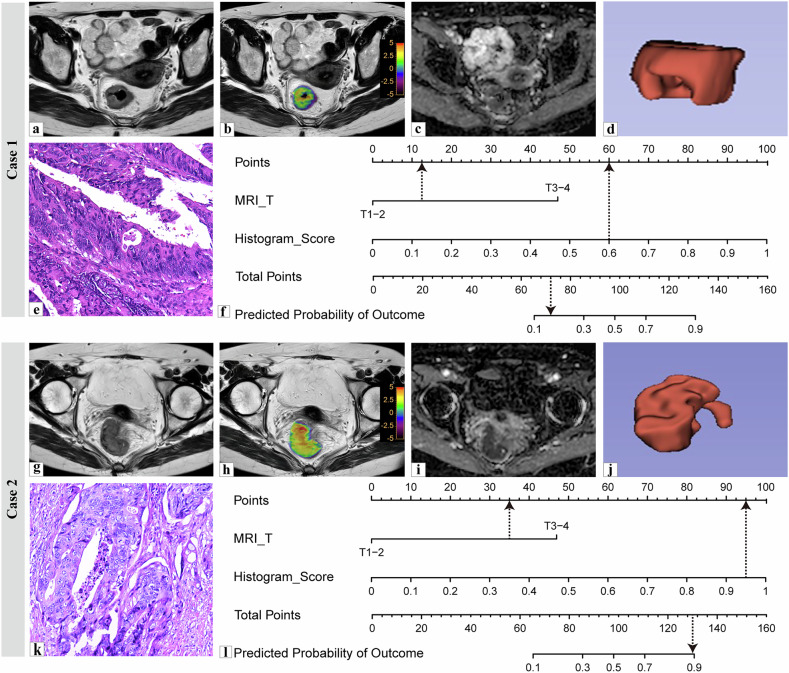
Nomogram method for calculating TB grade to predict the risk score of patients with RC. a–f Images from a 57-year-old woman with low-grade TB: (a) axial T2WI revealing diffuse thickening of the intestinal wall with a low signal; (b) APTw image fused with T2WI; (c) ADC image; (d) 3D VOI; (e) pathology image; and (f) nomogram indicating a risk score of 72, predicting a high-grade TB risk of 0.17. g–l Images from a 68-year-old woman with high-grade TB: (g) axial T2WI revealing diffuse thickening with low signal; (h) APTw image fused with T2WI; (i) ADC image; (j) 3D VOI; (k) pathology image; and (l) nomogram with risk score of 130, predicting a high-grade TB risk of 0.89. 3D, Three-dimensional; ADC, Apparent diffusion coefficient; APTw, Amide proton transfer-weighted; RC, Rectal cancer; ROI, Region of interest; TB, Tumor budding; T2WI, T2-weighted imaging
